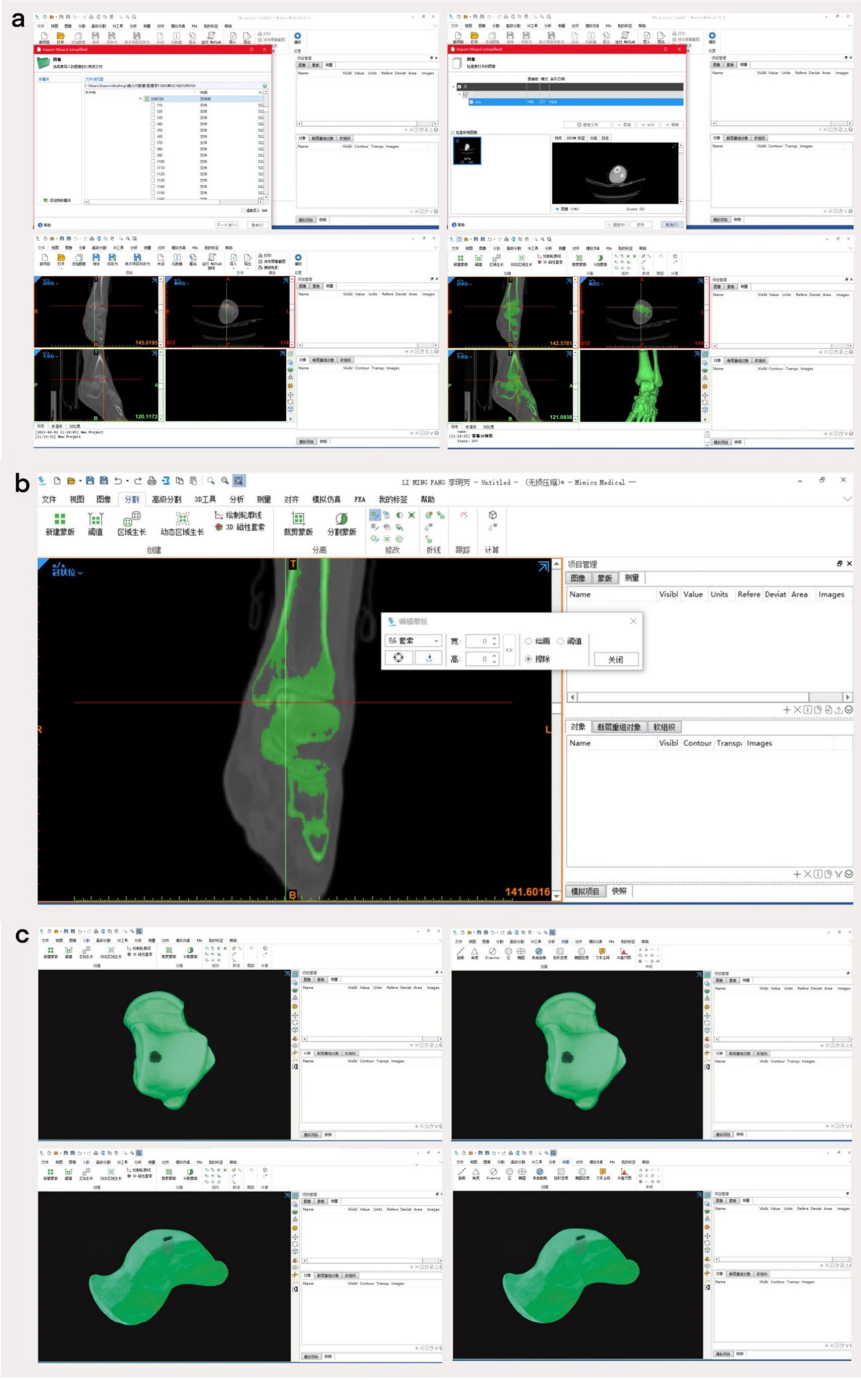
The 3D modeling procedure of the talus. a Import the CT data of the patient and reconstruct the 3D image of the ankle joint. b Extract the talus through the “Lasso” and “Erase” tools in the “Edit Mask” interface. c Fully display the precise location of the talus dome osteochondral injury in the top and medial views to measure the surface area and depth of the talus injury site
